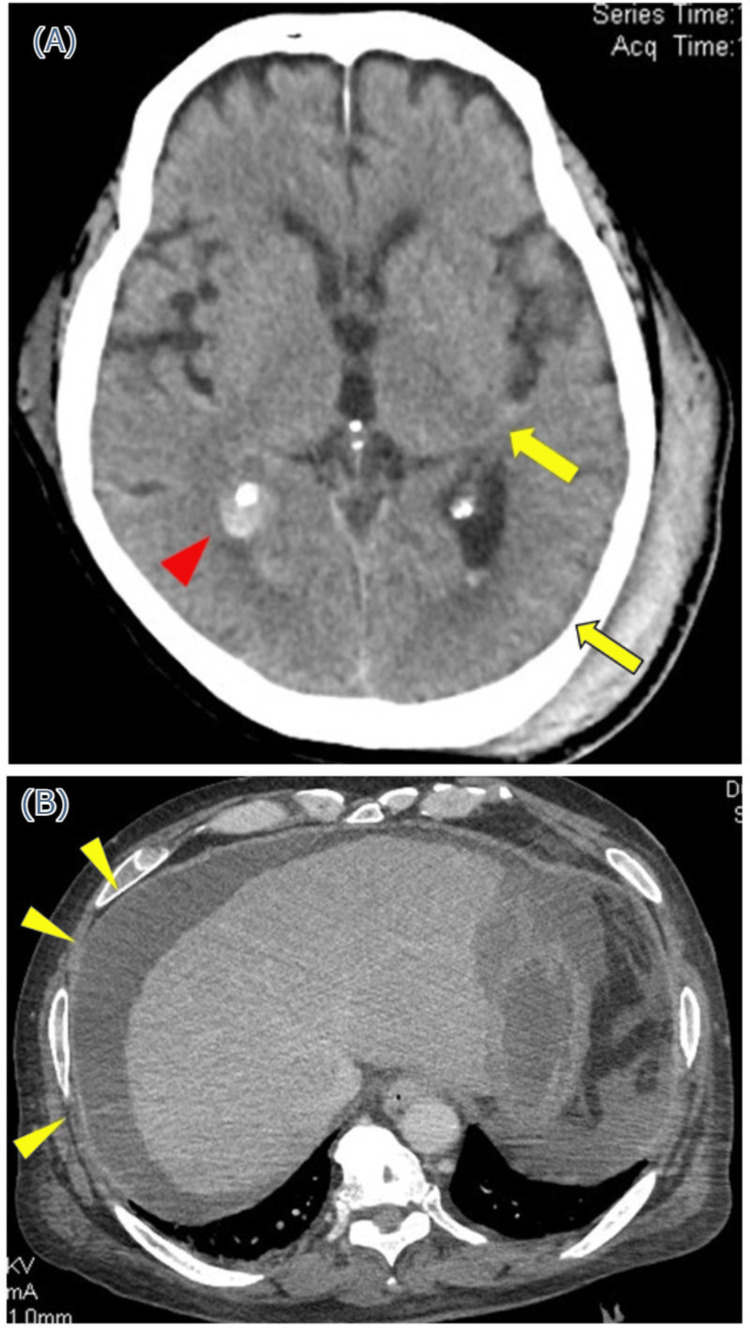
Computed tomography images (A) Computed tomography of the head reveals traumatic subarachnoid hemorrhage (yellow arrows) and hemorrhage in the right lateral ventricle (red arrowhead). (B) Computed tomography of the abdomen reveals ascites (yellow arrowheads) without other signs of infectious lesions.
